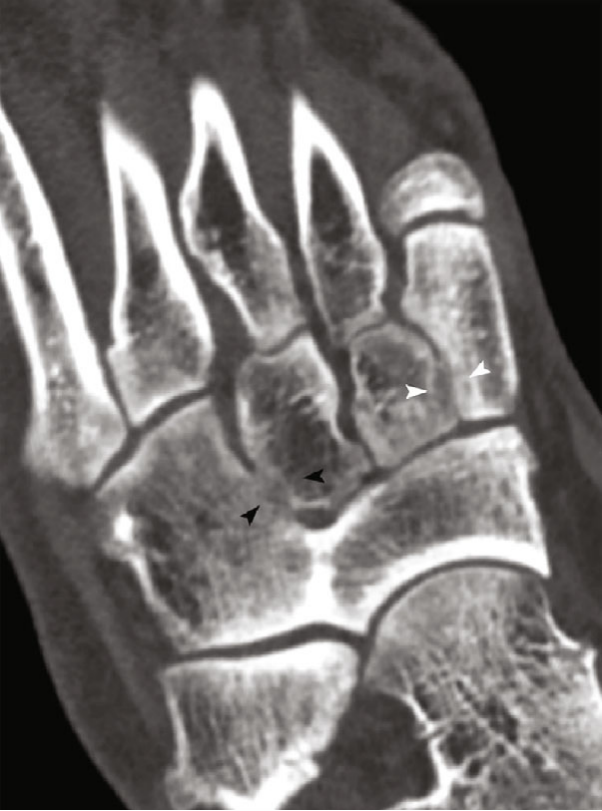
Oblique multiplanar reconstruction CT image of the right foot shows osseous cubonavicular coalition, associated with a partial osseous coalition between the cuboid and the third cuneiform with a focal lack of subchondral bone plate (black arrowheads) and bone irregularities between the first and second cuneiforms suggesting a nonosseous coalition (white arrowheads).
